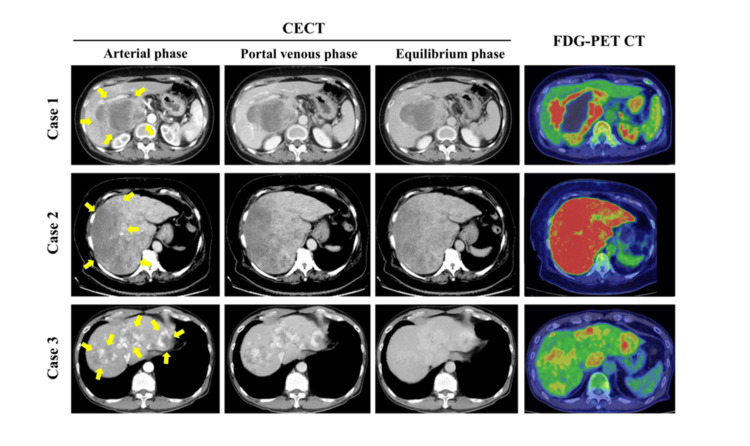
CT findings Dynamic contrast-enhanced computed tomography CT (CTCE) at the arterial phase, portal venous phase, and equilibrium phase are shown, as well as 18F-fluorodeoxyglucose positron emission tomography CT (FDG-PET CT) findings.
